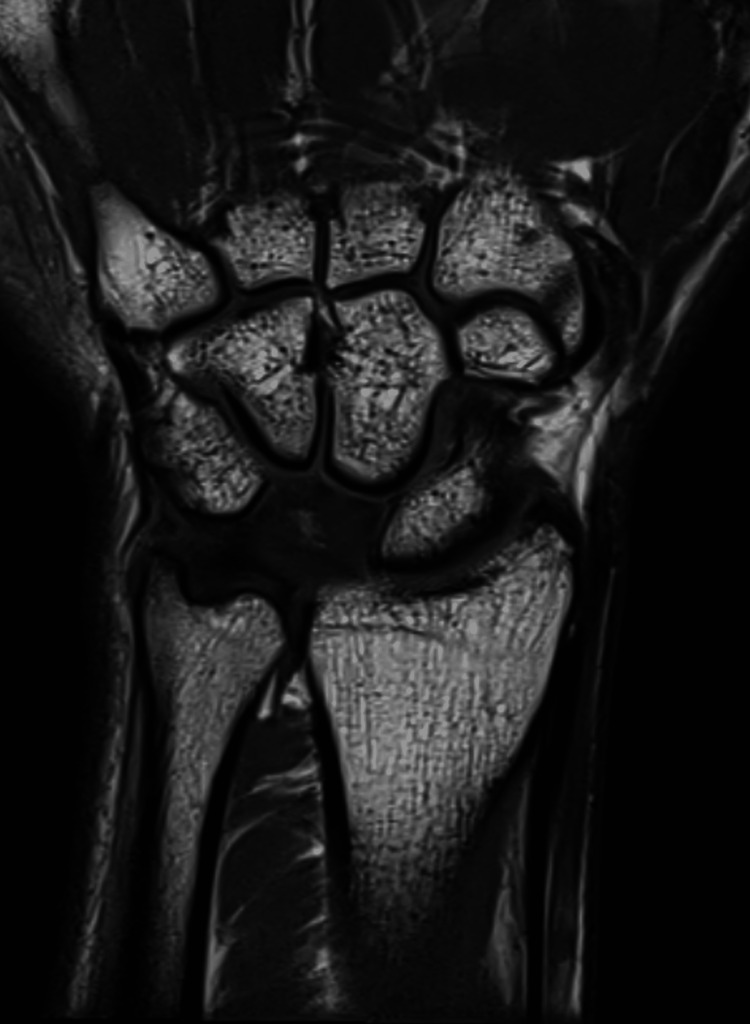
MRI showing avascular necrosis of the lunate
